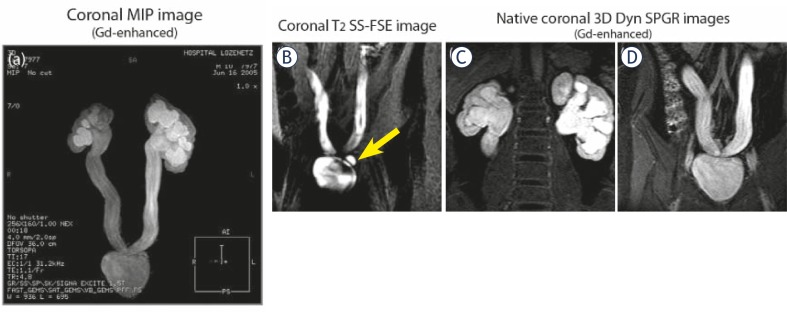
MR imaging of bilateral ureterocystoneostomies in 10-months old boy. A. Coronal post-contrast MIP image – persistent bilateral hydronephrosis and hydroureters following surgery. B. Coronal T2 SS-FSE image – a bladder diverticula. C,D. Post-contrast coronal 3D Dyn SPGR image on the kidney level (C) and on the level of the bladder (D) – re-implanted dilated hydroureters.
